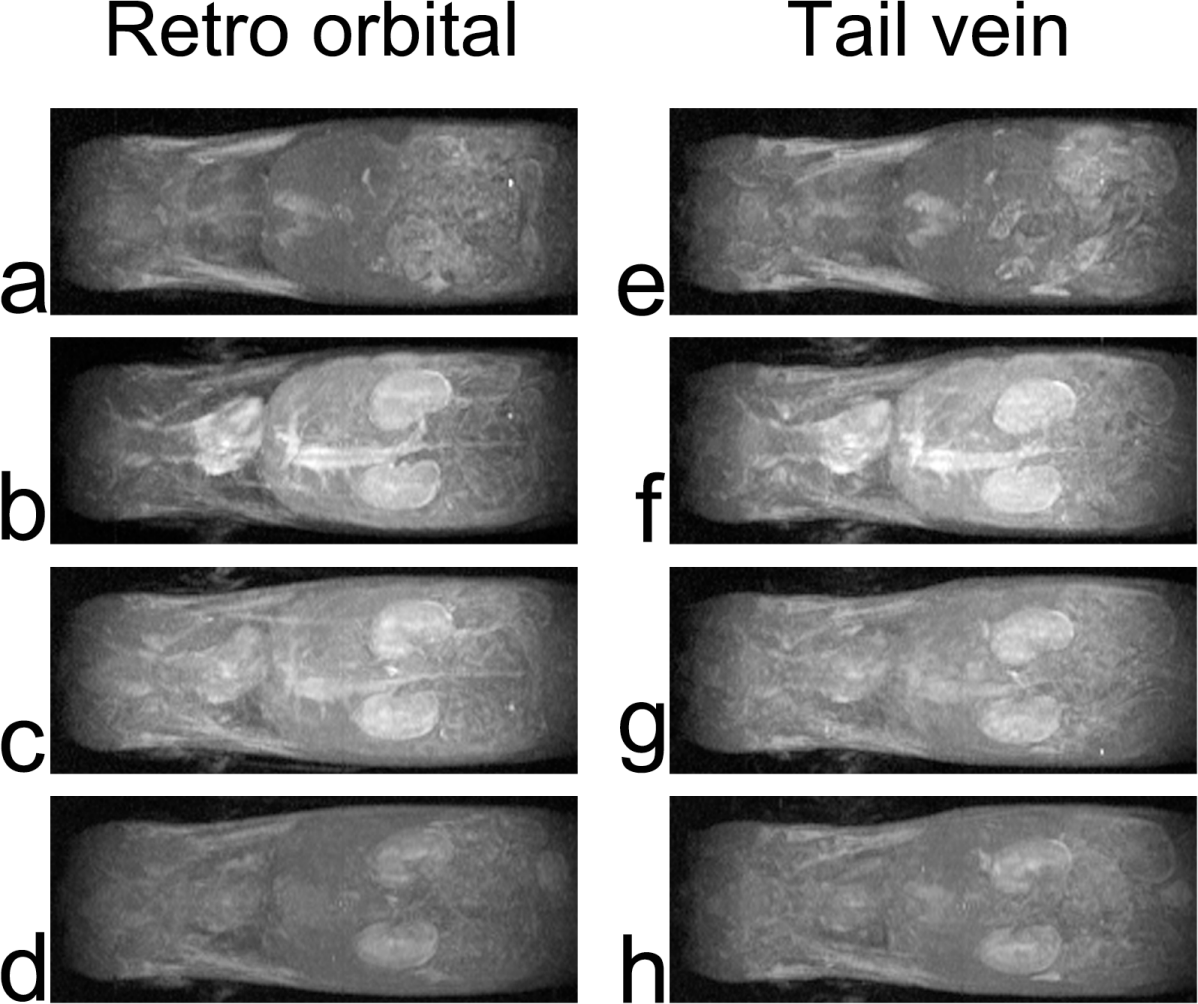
Partial maximum intensity projections of thoracoabdominal MR images. Left column, from top to bottom, images obtained before (a), 30 s (b), 3 min 30 s (c), and 10 min (d) after delivering the contrast agent via the retro-orbital route. Right column, images obtained before (e), 30 s (f), 3 min 30 s (g), and 10 min (h) after delivering the contrast agent via the tail vein route.
